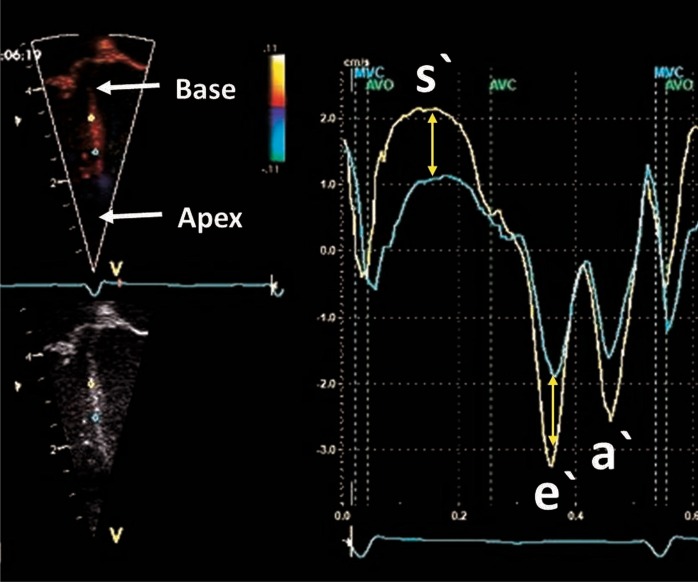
Difference in velocity between two points along the long axis of the septum. The curves show tissue velocities by tissue Doppler during the cardiac cycle. The point closer to the base (yellow) has a higher systolic and diastolic velocity when compared with the point closer to the base (green). The difference in velocity is used to calculate strain rate and derive strain of that segment bordered by the two points
